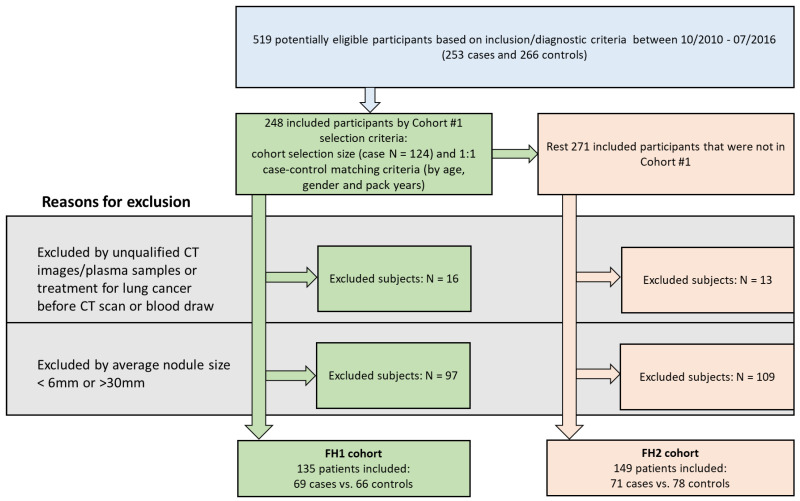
Study flow of participants. A total of 519 qualified subjects were enrolled. A total of 248 were included in Fred Hutch (FH) 1 cohort by selecting 124 cases and case–control matching 1:1 on age, gender, and pack years. The remaining 271 were evaluated for inclusion in FH2 cohort. In FH1, 113 were excluded due to unqualified CT/plasma or CT/plasma prior to treatment or nodule sizes, leaving 135 total subjects in FH1 with indeterminate pulmonary nodules. In FH2, 149 patients were included after excluding 122 subjects.
